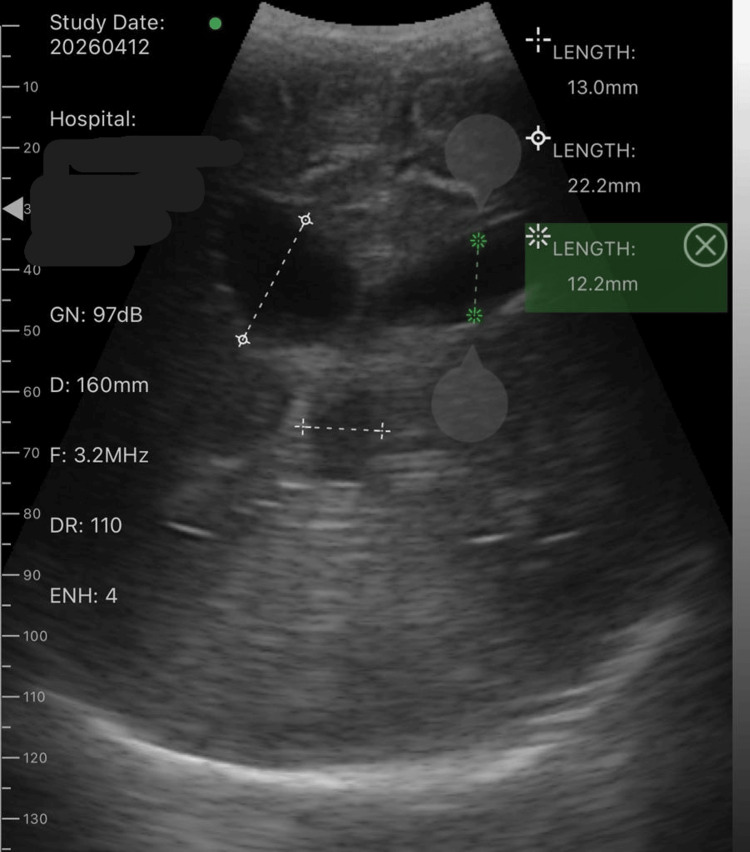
Bedside cranial ultrasound images. Coronal view demonstrating dilated lateral ventricles.
